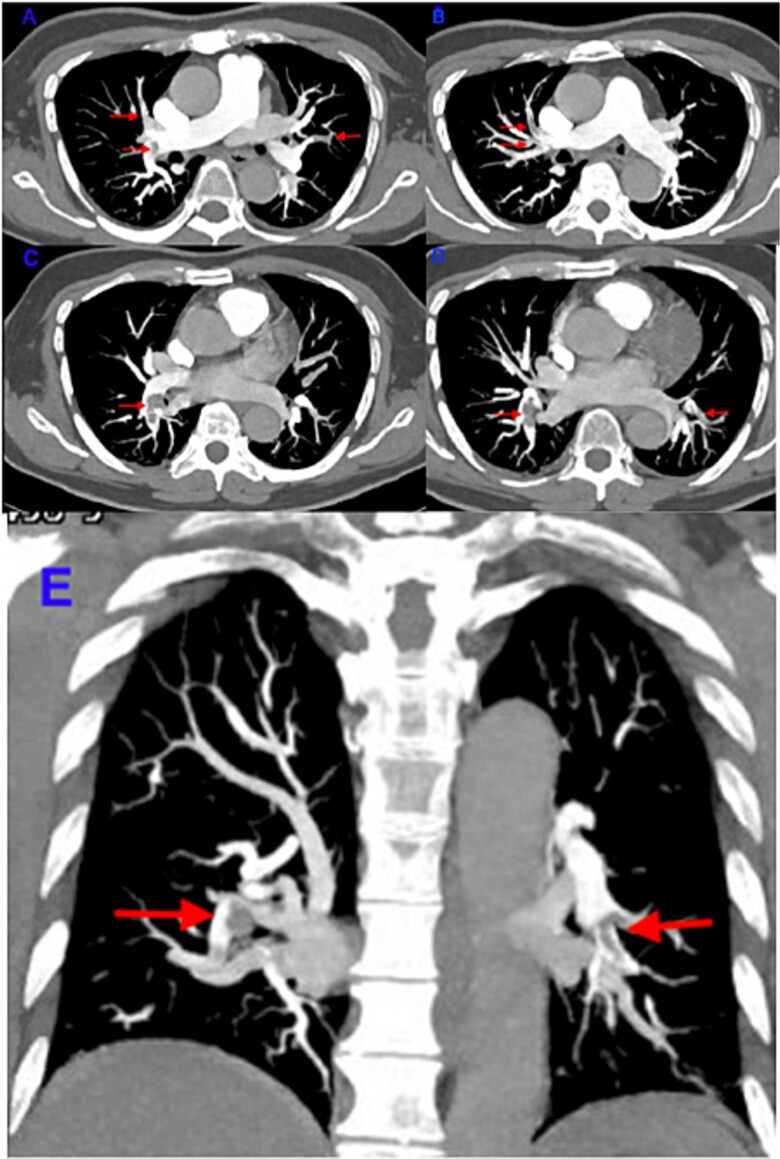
(A,B,C,D,E) shows filling defects in CT pulmonary angiogram.
